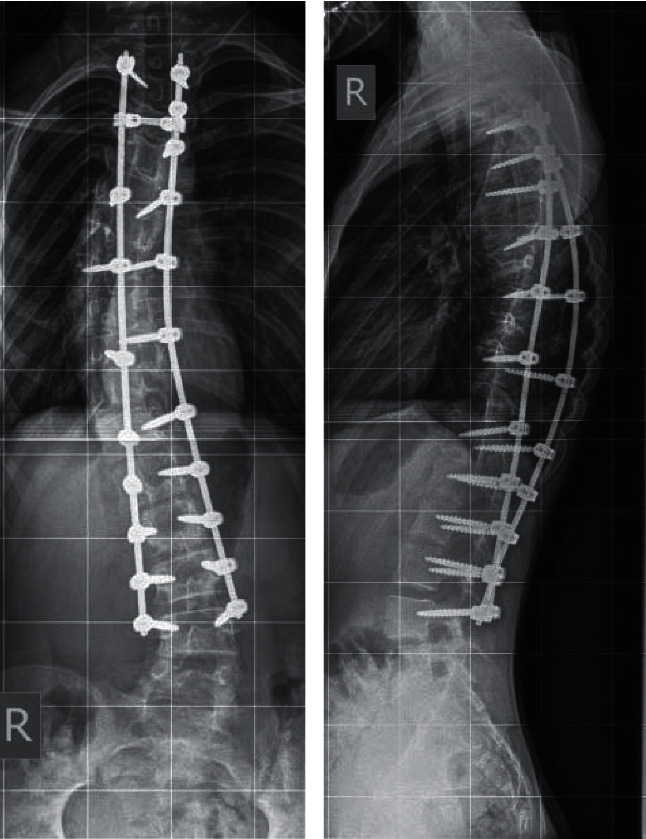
Anteroposterior and lateral X-rays demonstrating scoliosis correction with posterior instrumentation.
